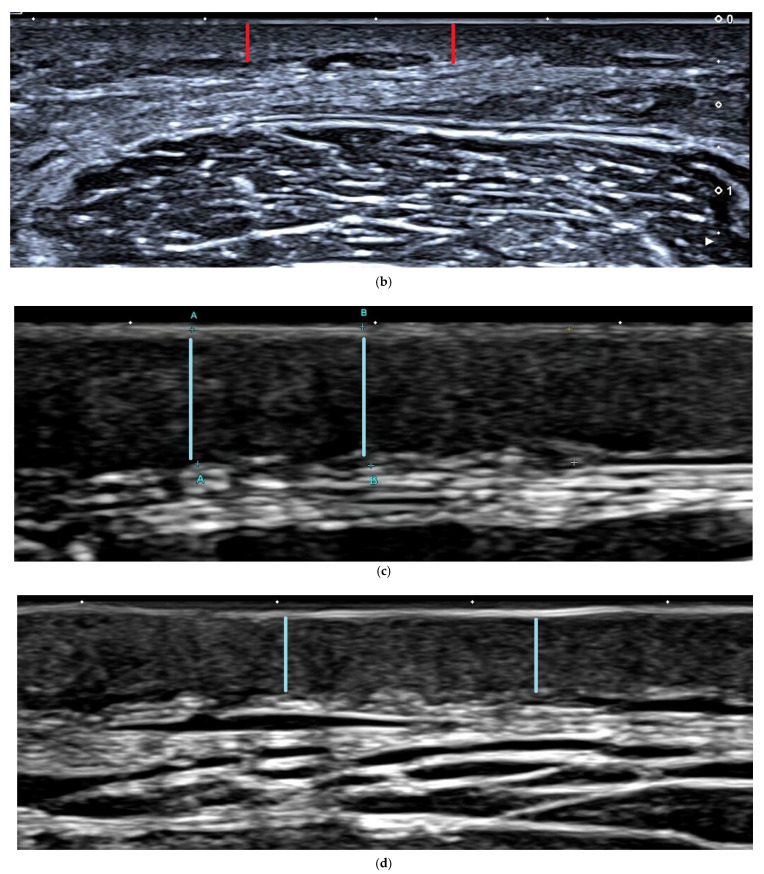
Ultrasonic images of patients four weeks before and after treatment. (a) AFL+MTX group patient 4 weeks before treatment. The uppermost layer, the epidermis layer, is hyperechoic (shown with white arrows), and the underlying dermis is hypoechoic (shown with red line) and indistinguishable from the fat layer. (b) AFL+MTX group patient 4 weeks after treatment. The dermis is thinner than before (shown with red line) and can be easily distinguished from the fat layer. (c) AFL group patient 4 weeks before treatment. The dermis (shown with blue line) is indistinguishable from the fat layer. (d) AFL group patient 4 weeks after treatment. The dermis is thinner (shown with blue line) than before and clearly identified with the fat layer.
